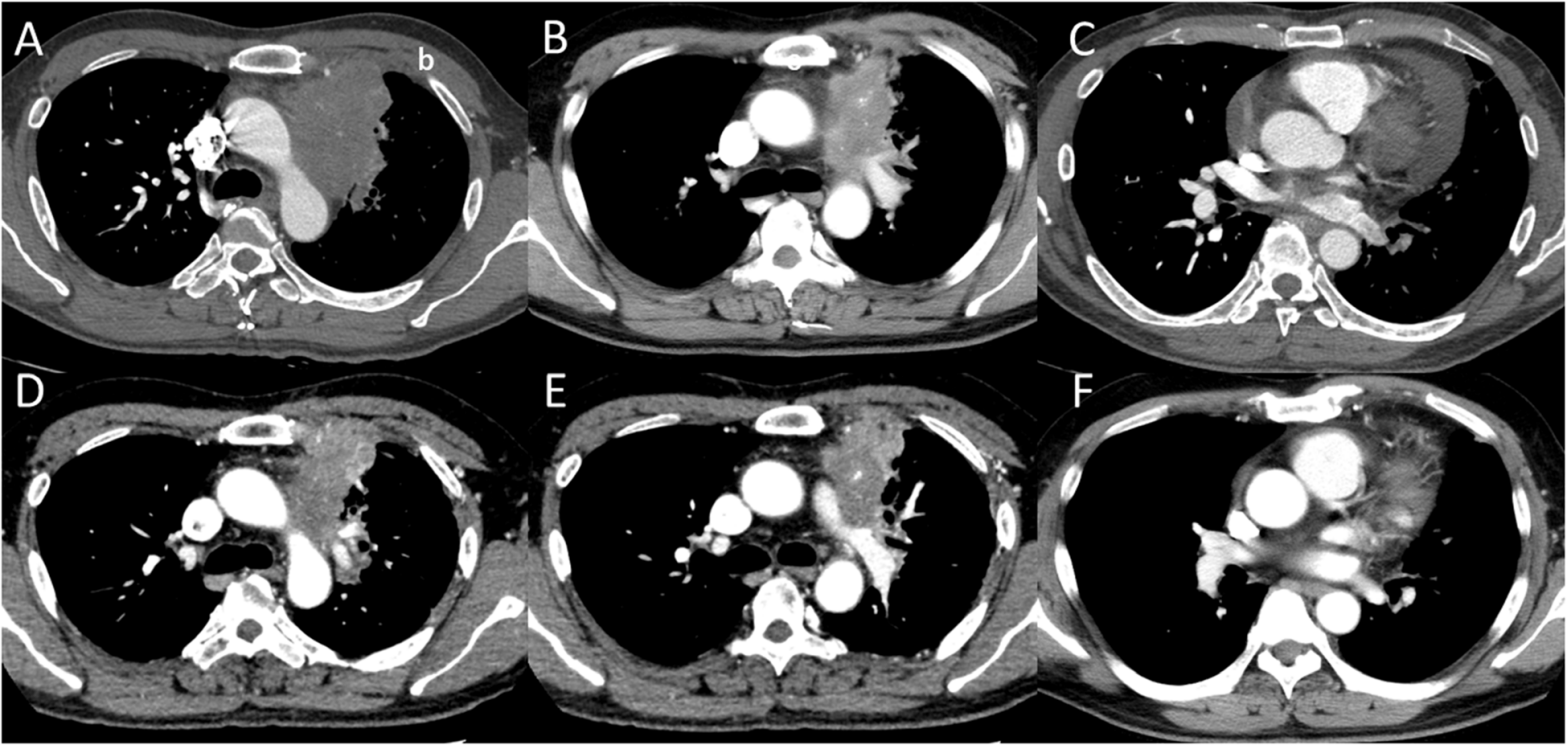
Radiographic images obtained (a-c) before and (d-e) after chemotherapy. Chest computed tomography (CT) findings showed an anterior mediastinal tumor invading the (a) aortic arch and (b) left hilar part of the left lung, as well as (c) pericardial effusion. After the end of the course of chemotherapy, CT showed that the tumor had shrunk, though invasion to (d) the aorta and (e) left pulmonary artery was still evident, while (f) pericardial effusion had disappeared. Lt PA, left pulmonary artery
